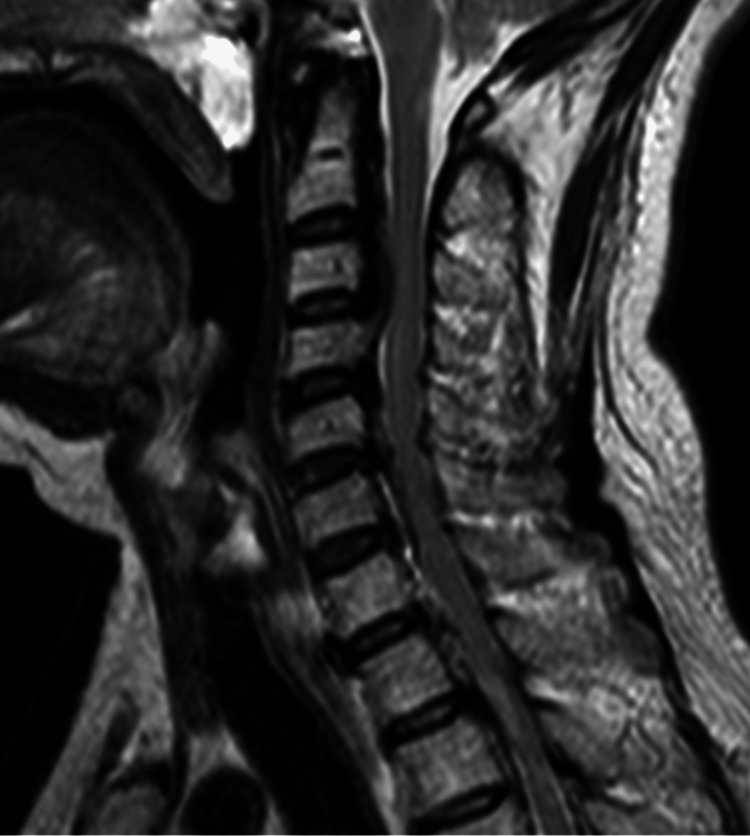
Cervical spine magnetic resonance imaging(MRI) showed spinal canal stenosis at C3 or lower and maximum stenosis at C6/7.
